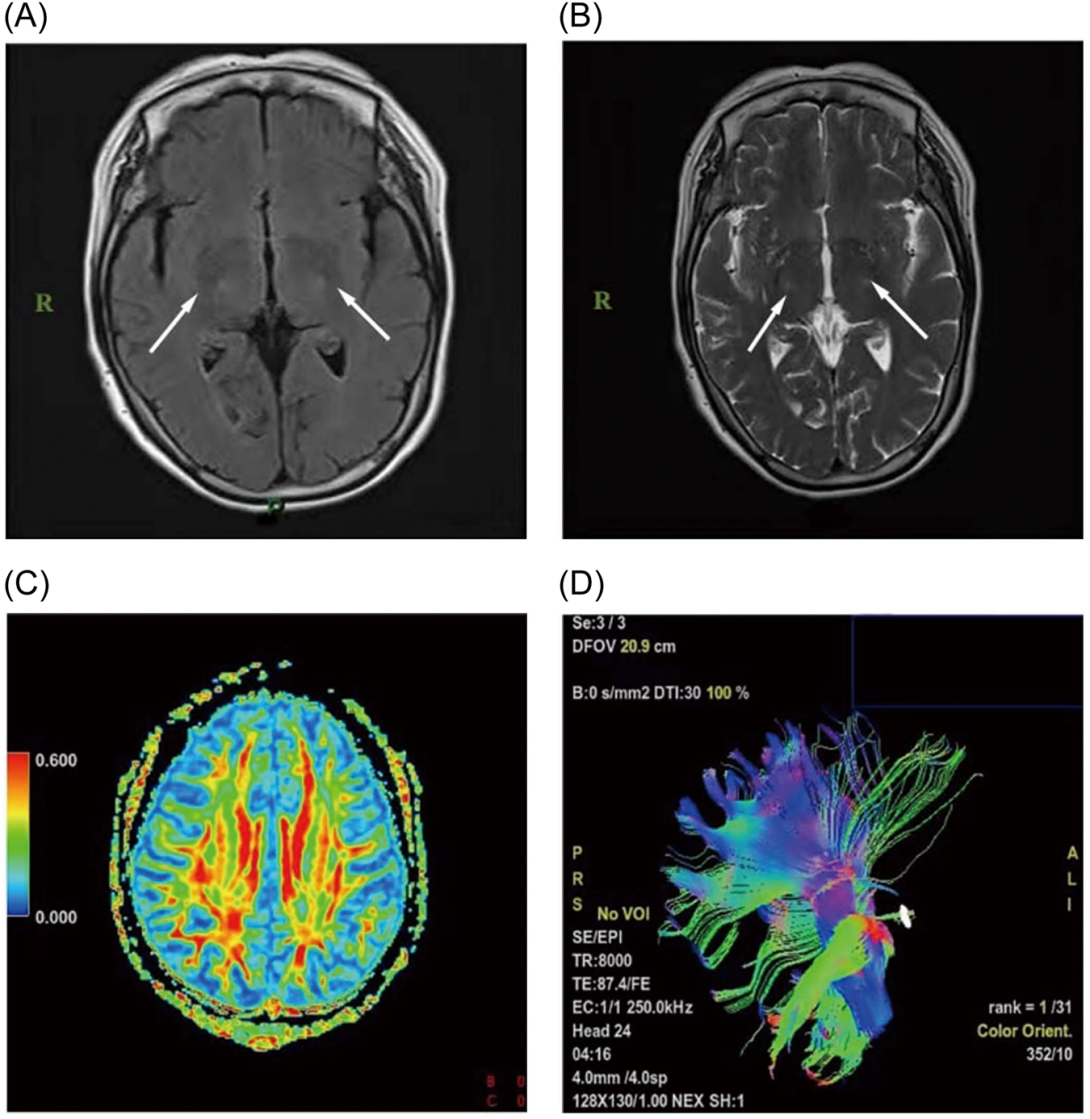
Brain magnetic resonance imaging. (A) T1‐weighted imaging. (B) T2‐weighted imaging. The arrows in (A) and (B) show bilateral corticospinal tract abnormalities. T Panels (C) and (D) are brain MRI nerve fiber bundle angiography, and no abnormal manifestations were shown in the figure. MRI, magnetic resonance imaging. [Color figure can be viewed at wileyonlinelibrary.com] [Correction added on 24 July 2025, after first online publication: The figure 2 was revised to improve the clarity by adding the arrows.]
